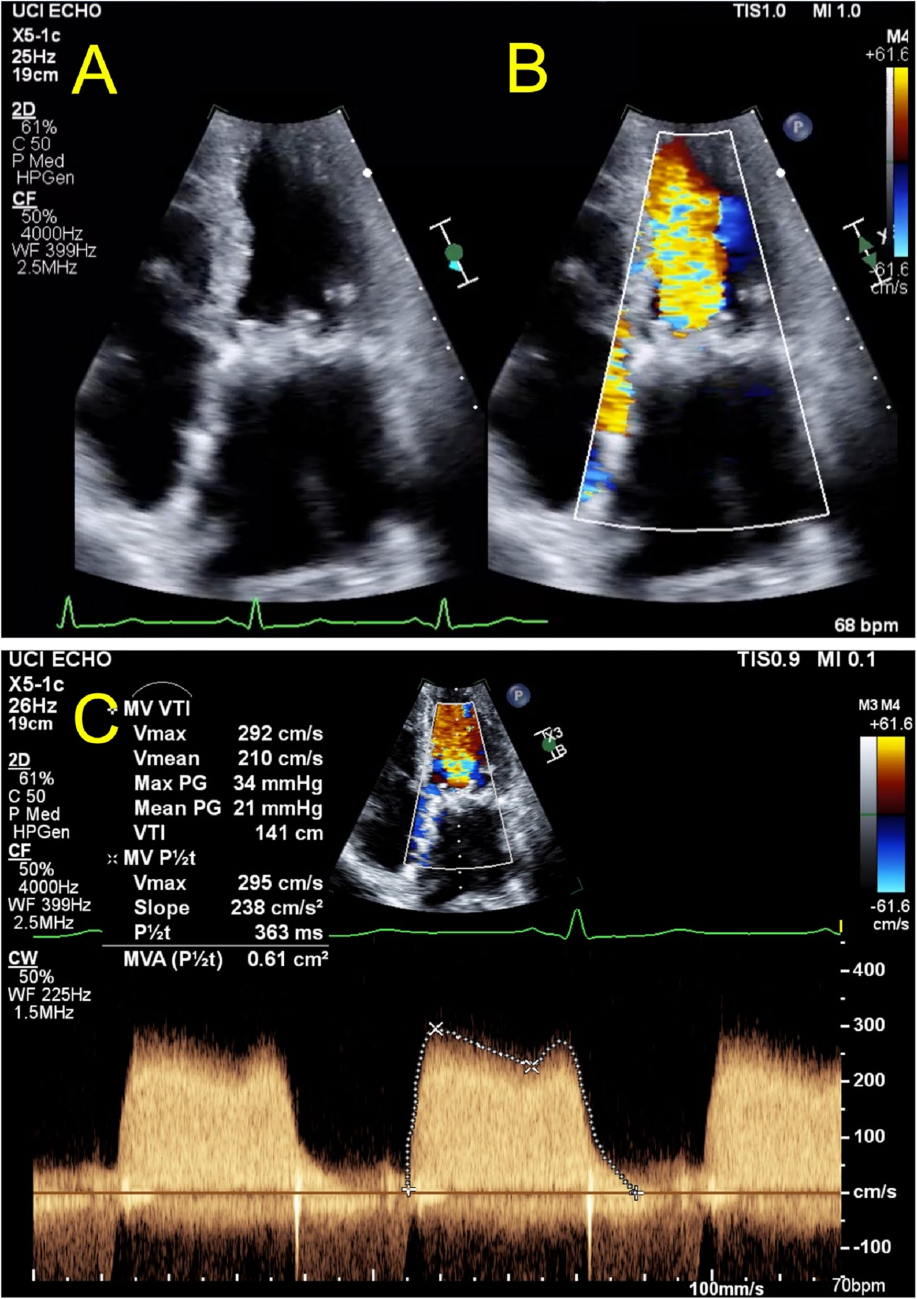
Pre-thrombus echocardiographic images of apical 4 chamber view of bioprosthetic mitral valve. Pre-thrombus transthoracic echocardiogram (TTE) and images of the bioprosthetic mitral (MV) valve in end-diastole demonstrating severely thickened and restricted leaflet motion in the (A) apical 4 chamber view without color Doppler, (B) apical 4 chamber view with color Doppler showing flow acceleration at the level of the MV annulus and (C) continuous wave Doppler tracing with dense envelope with mean gradient of 21 mmHg by velocity-time-integral at a heart rate of 70 beats per minute
